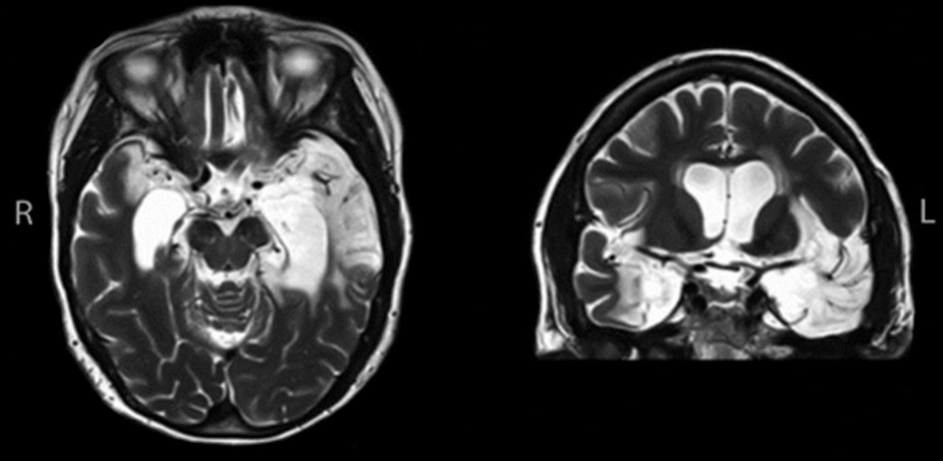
High resolution magnetic resonance images showing the extent of temporal lobe damage in patient LSJ. See text for description of lesion.
